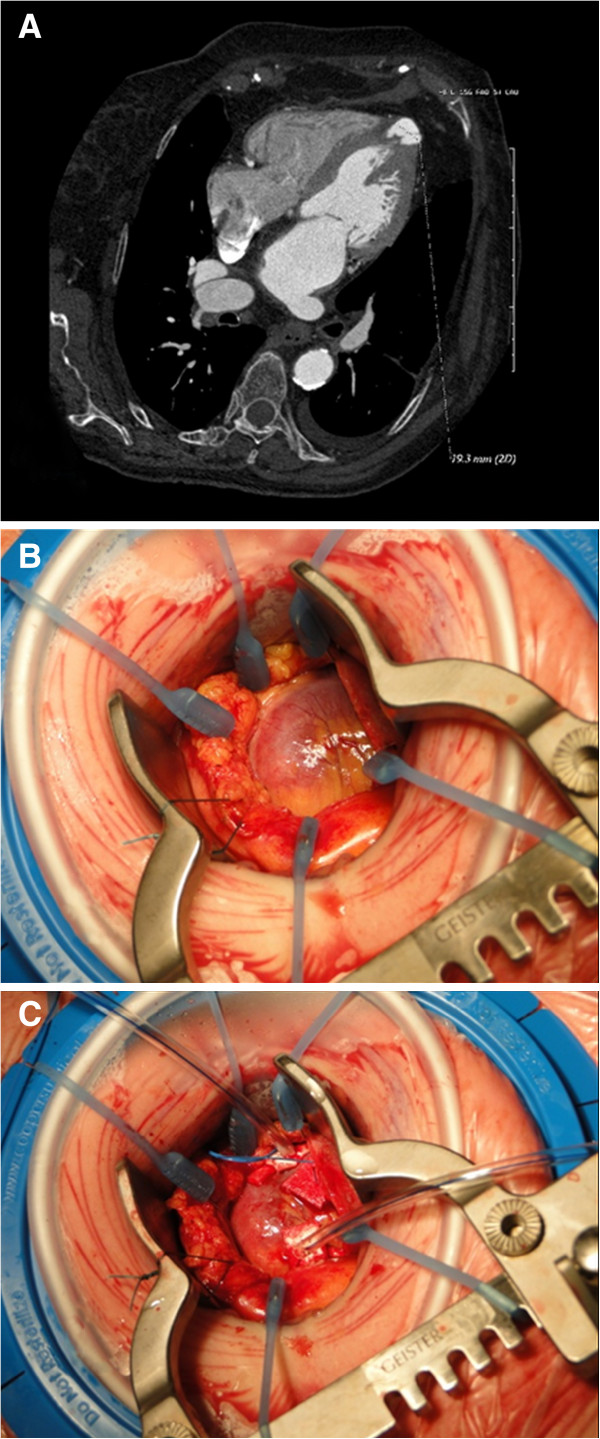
A) A computed tomography scan showing the left ventricular apical diverticulum: note the absence of thrombi. B) Intraoperative view of the cardiac apex: macroscopically, there is no evidence of a diverticulum, whereas the palpation reveals a soft portion. C) Careful preparation of a double pledgeted 3–0 Prolene purse-string suture around the diverticulum.
